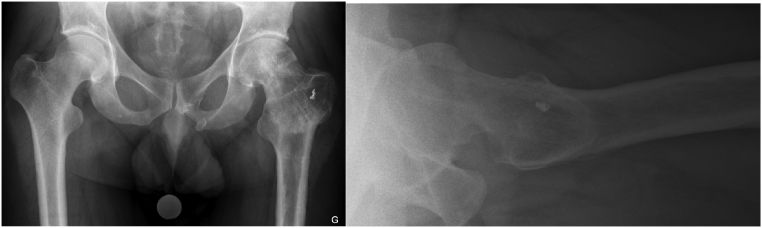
AP pelvis and cross-table lateral hip radiographs after hardware removal of the left hip.
